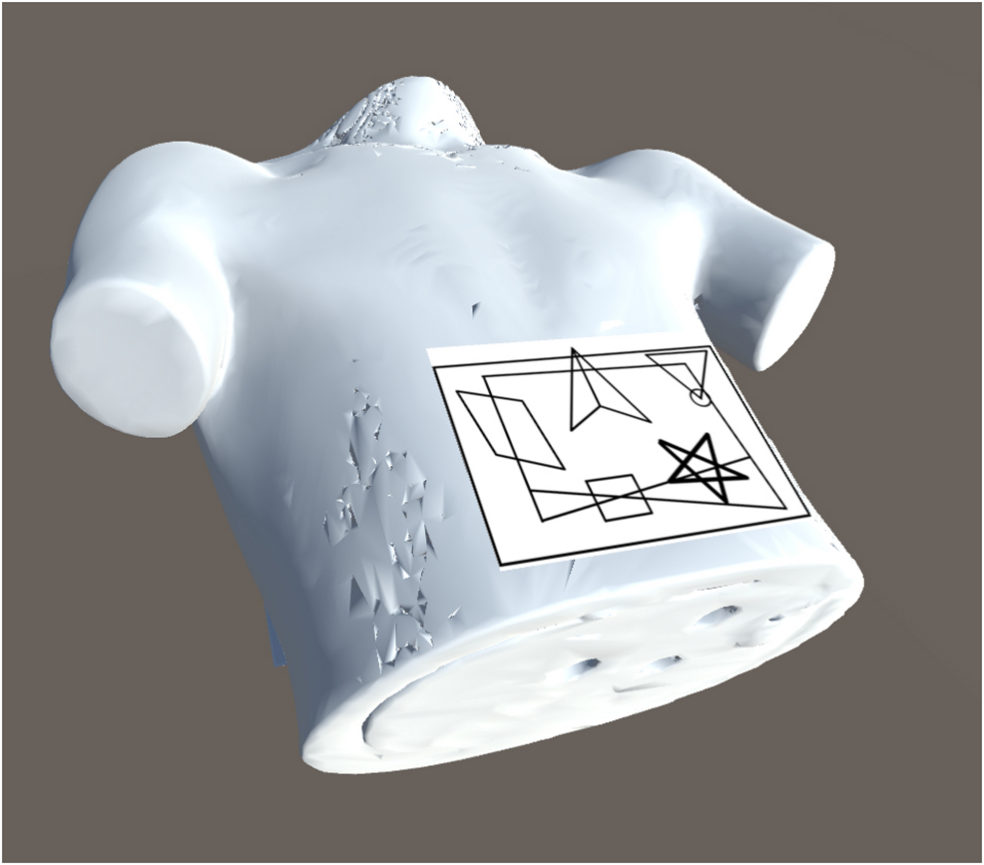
3D rendered chest CT images and the virtual recognition marker in Unity
